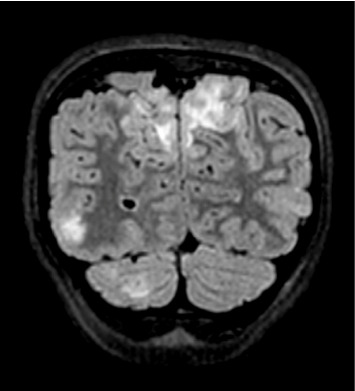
Coronal MRI T2-FLAIR sequence with hyperintensities in bilateral parietal–occipital lobes, right inferior occipital lobe, and right cerebellar hemisphere.
